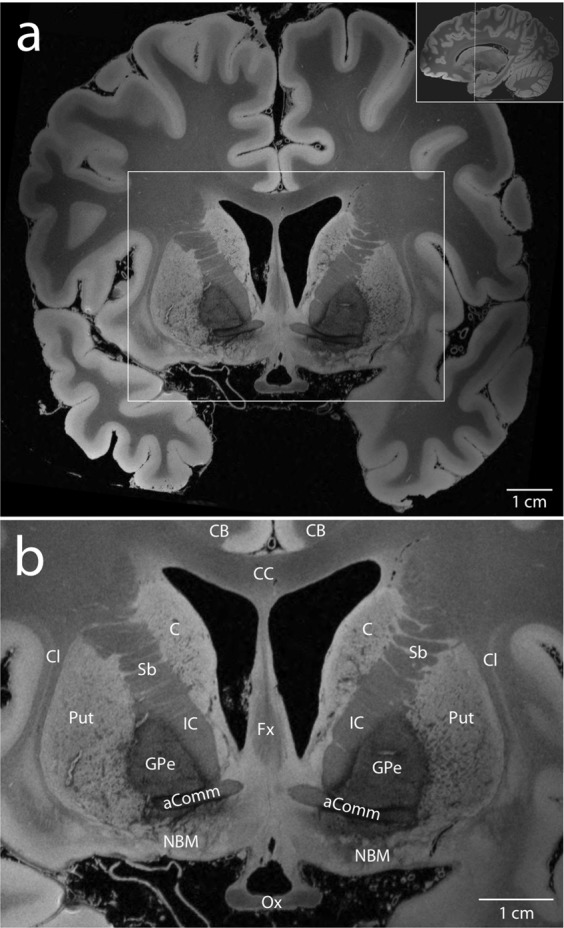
Delineation of basal ganglia and basal forebrain neuroanatomy. A representative coronal section from the synthesized FLASH25 volume is shown in the plane of the anterior commissure (aComm; see inset in a). A zoomed view of the basal ganglia and basal forebrain (within the white rectangle in a) is shown in (b). The anatomic detail that can be visualized in this *ex vivo* 100 μm resolution MRI dataset is beyond that which can be seen in standard *in vivo* MRI datasets. Neuroanatomic abbreviations: C = caudate; CB = cingulum bundle; CC = corpus callosum; Cl = claustrum; Fx = fornix; GPe = globus pallidus externa; IC = internal capsule; NBM = nucleus basalis of Meynert; Ox = optic chiasm; Put = putamen; Sb = striatal bridges.
